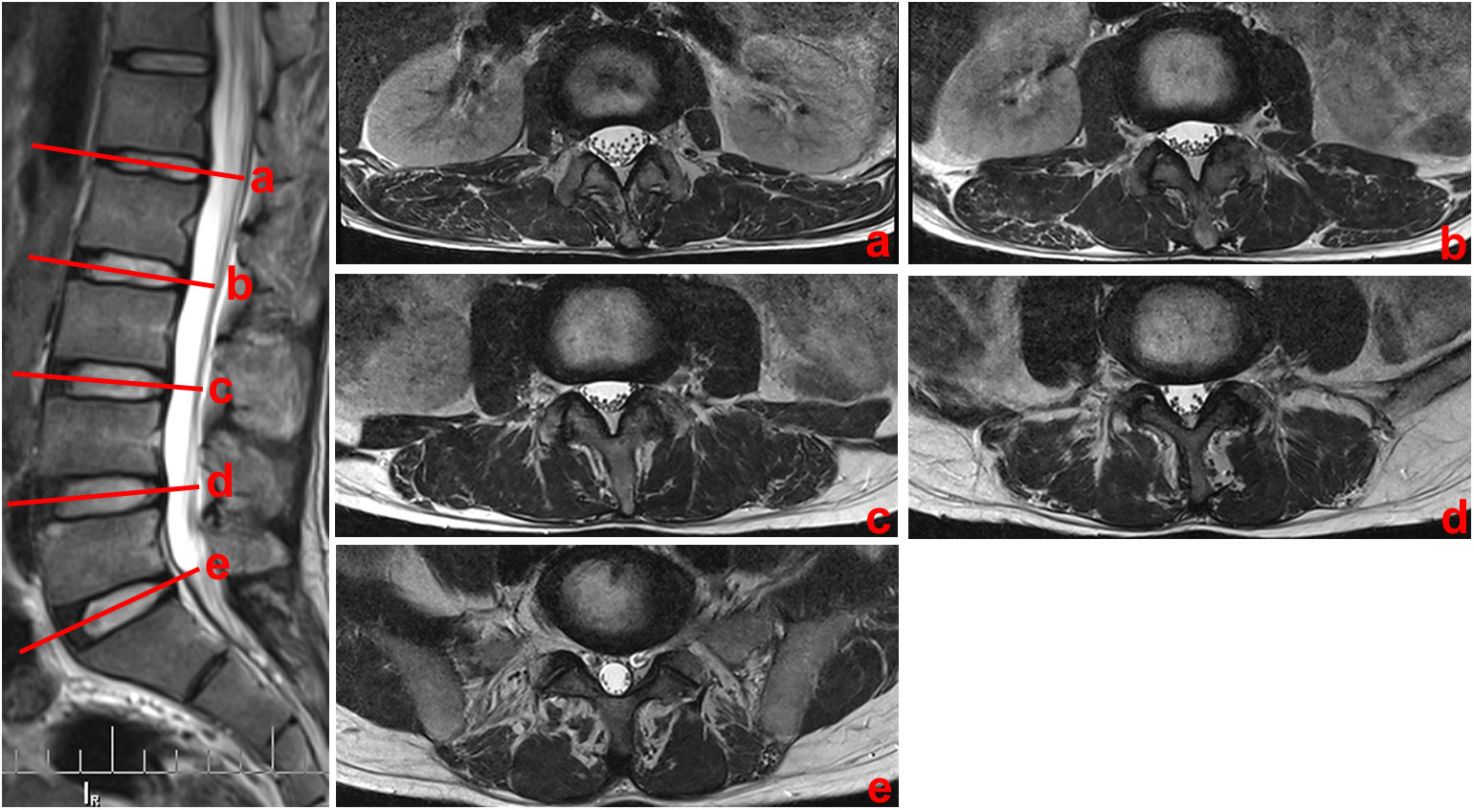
Transverse MR images at different disc level. (a) L1-L2 intervertebral disc level; (b) L2-L3 intervertebral disc level; (c) L3-L4 intervertebral disc level; (d) L4-L5 intervertebral disc level; (d) L5-S1 intervertebral disc level
